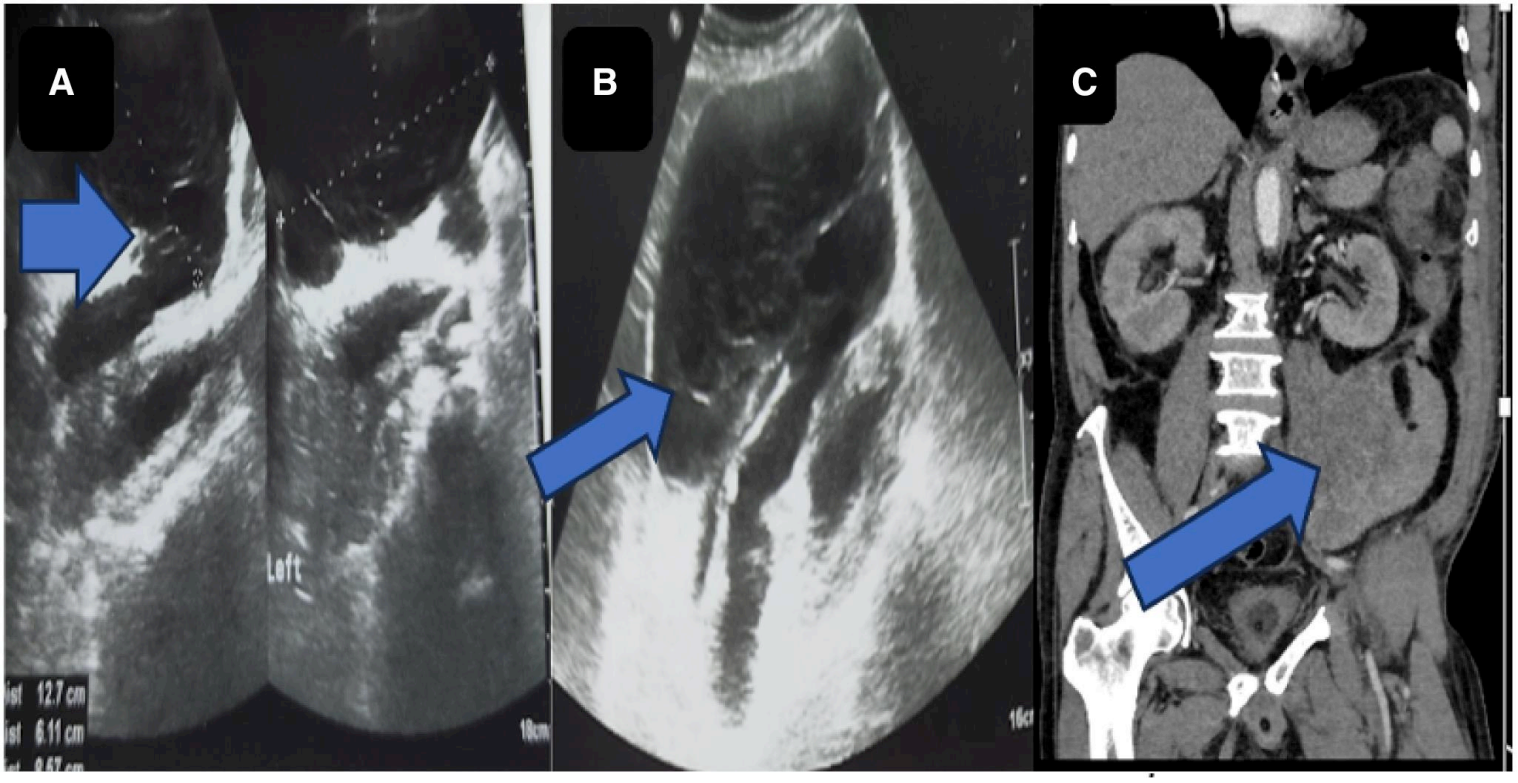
Ultrasound images (A, B) showing well-defined hypodense collection measuring 12.7 × 6.1 × 8.5 cm with echogenic contents and multiple strands in the left psoas muscle suggestive of intra muscular hematoma. Coronal sections of contrast-enhanced CT abdomen (C) showing large hematoma involving the intramuscular plane of posterolateral aspect of psoas muscle.
